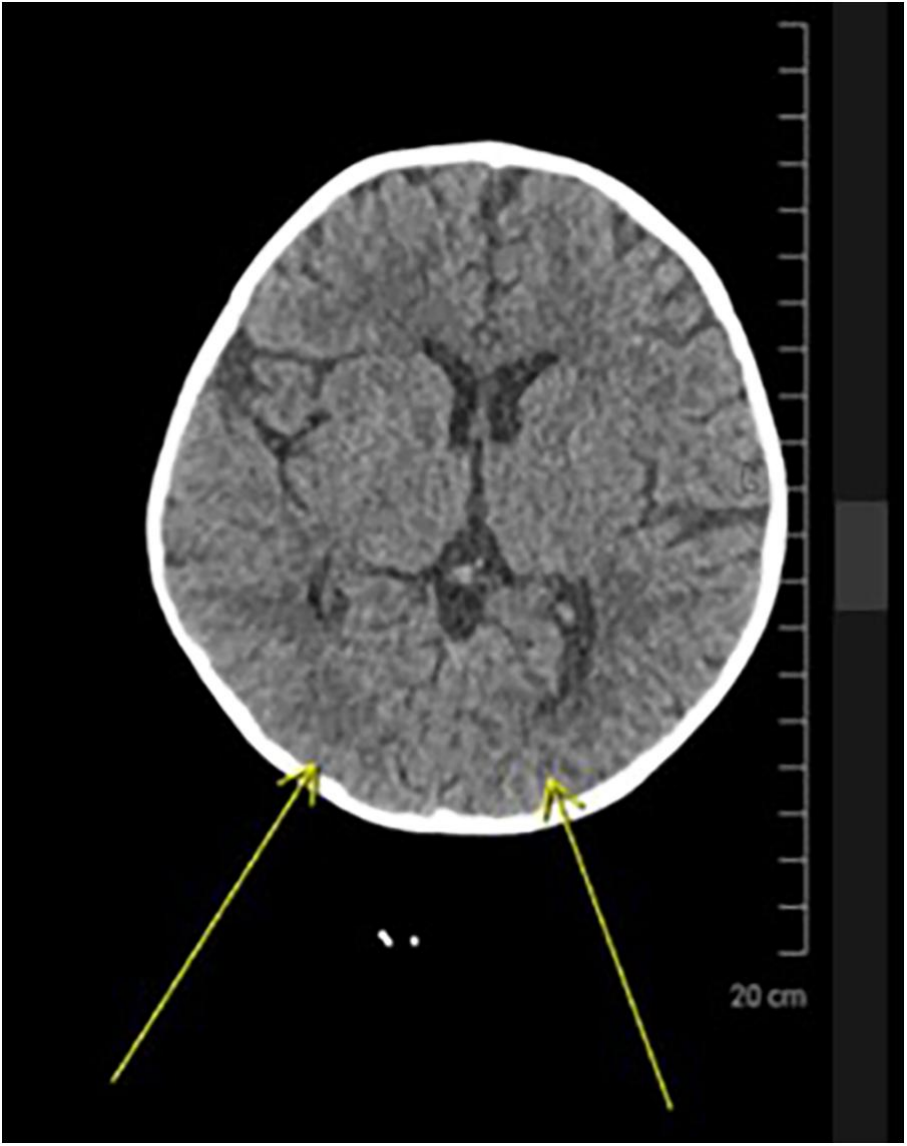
Axial brain CT showing hypodensity in the right occipital lobe (yellow arrows), consistent with embolic infarction. The revised annotation accurately indicates the infarct location.
